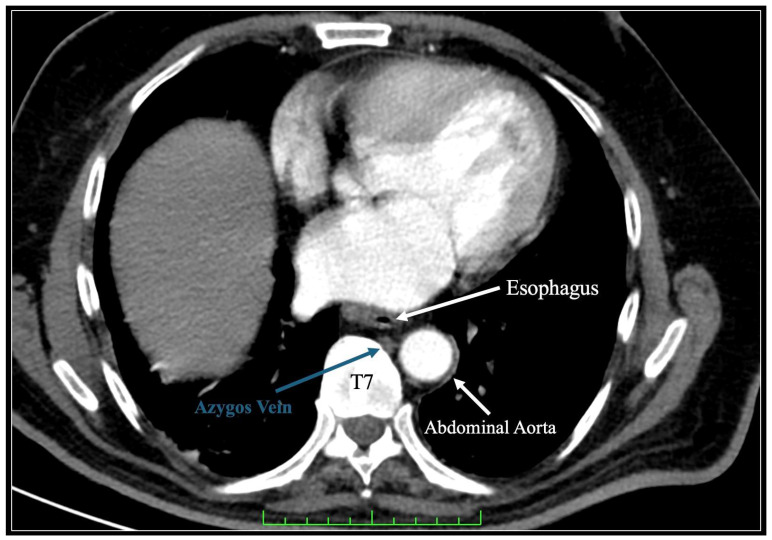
Computed tomography angiography of azygos vein, abdominal aorta and esophagus demonstrated in axial reconstruction.
